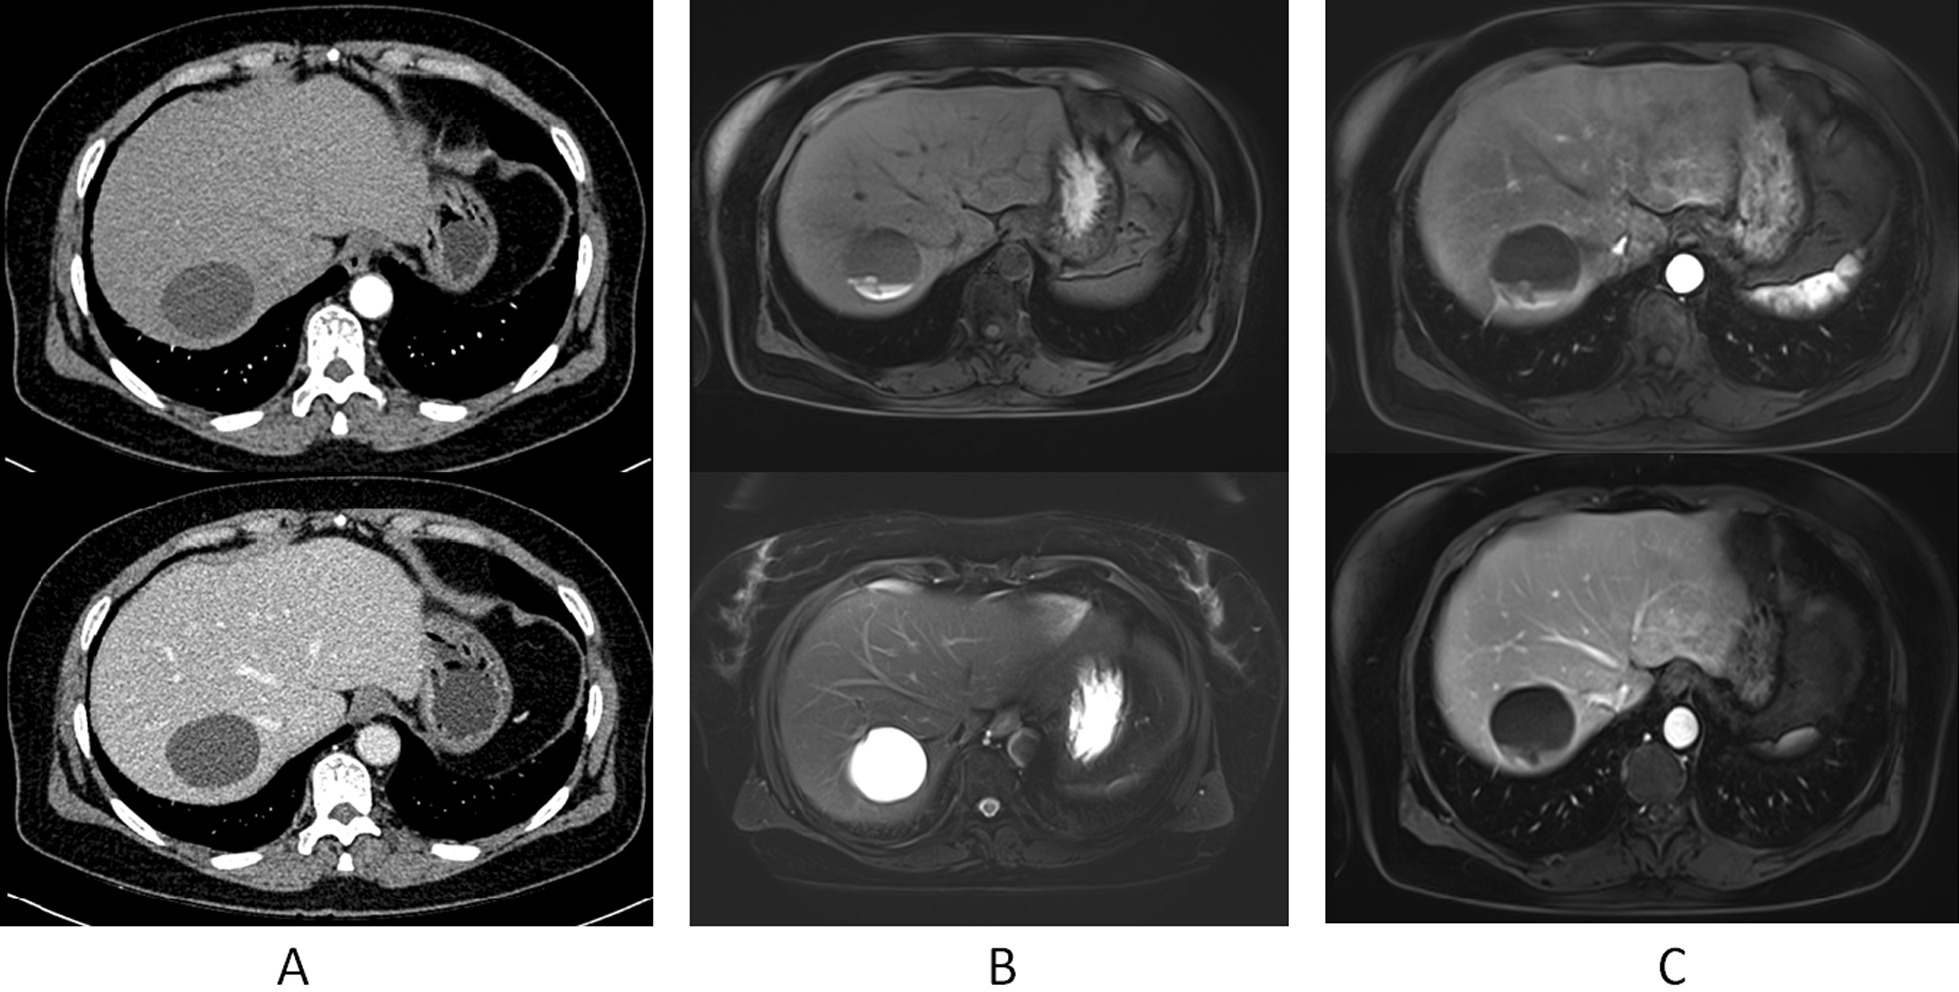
Preoperative imaging examination. A Upper abdominal enhanced computed tomography. B Hepatobiliary T1, T2 magnetic resonance imaging. C Hepatobiliary enhanced MRI
